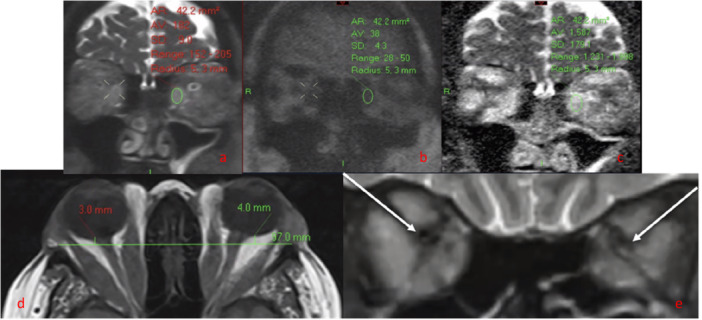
Coronal non‐EPI HASTE DWI MRI images ((a) = b0, (b) = b1000, (c) = ADC map) in a patient with severe TED. A region of interest has been placed in the left medial rectus muscle belly, showing markedly elevated ADC (1587) on (c). (d) Axial MRI T1 image, demonstrating radiological measurement of proptosis‐ the distance between the inter‐zygomatic line and the posterior globe in a TED patient (Normal range: 5.00–10.00 mm posterior to the inter‐zygomatic line [16, 17]). (e) Coronal MRI STIR image showing apical crowding (arrow) of the optic nerves bilaterally in a patient with TED and DON.
